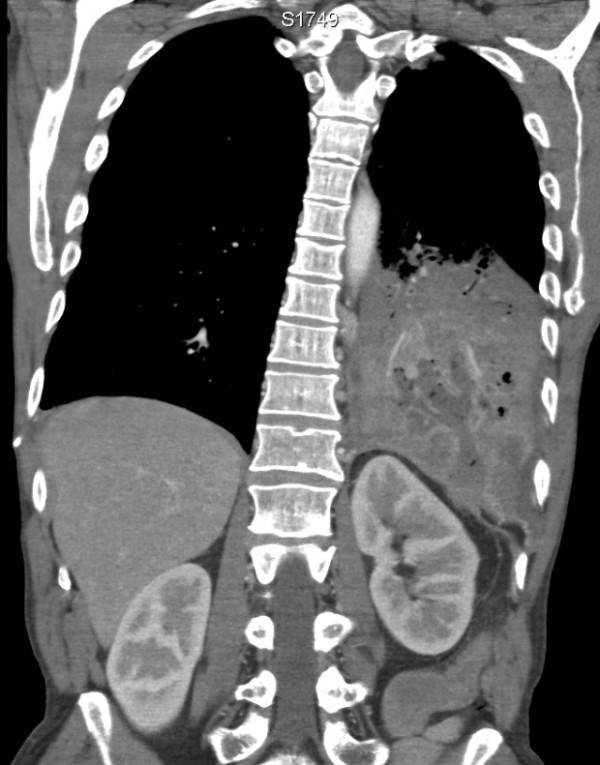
Coronal CT image shows the cavitating left lower lobe pneumonia. The fluid- and gas-containing collection transgresses the diaphragm and enters the retroperitoneal space. Although not shown here, this collection is contiguous with an abnormally thickened splenic flexure.
